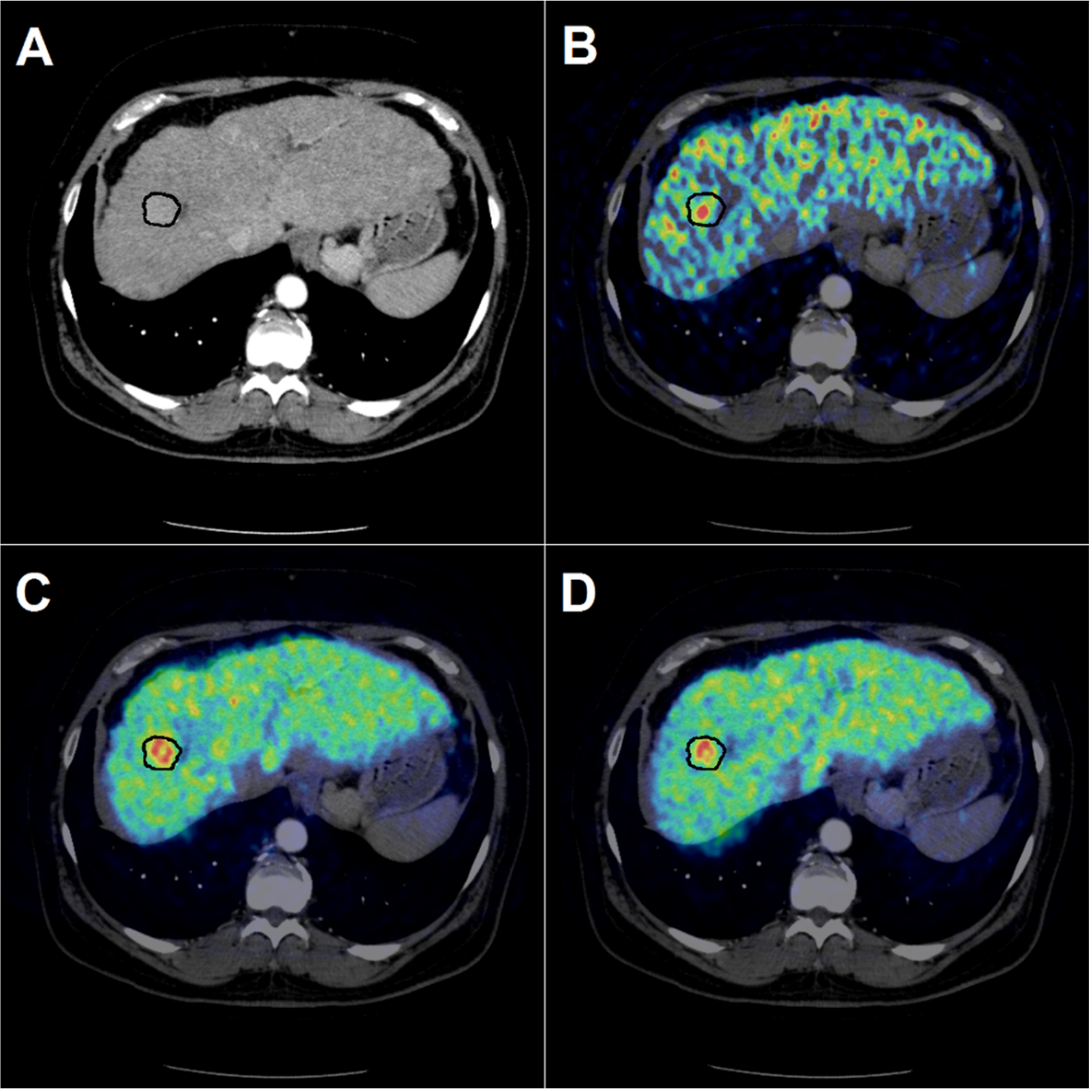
Contrast-enhanced CT image (arterial phase) with VOI-definition around the hypervascular HCC tumour (a) fused with the metabolic PET image (b), with the 1-h static image (c), and with the 2-h static image (d)
